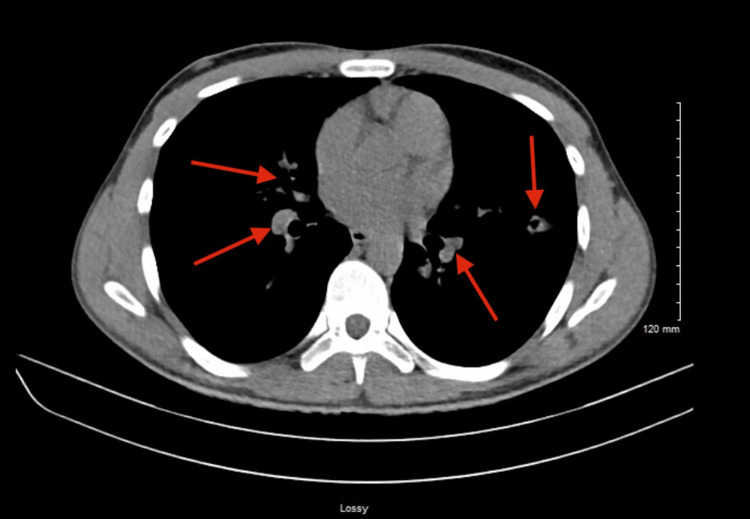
Non-contrast chest computed tomography (CT) demonstrating multilobar cavitary disease. Selected axial lung-window CT image demonstrates multiple air-containing cavities within areas of abnormal lung parenchyma, predominantly in the right upper lobe, with surrounding nodular consolidation (red arrows). Additional cavitary disease was present in the left upper and lower lobes on adjacent images. CT: computed tomography.
